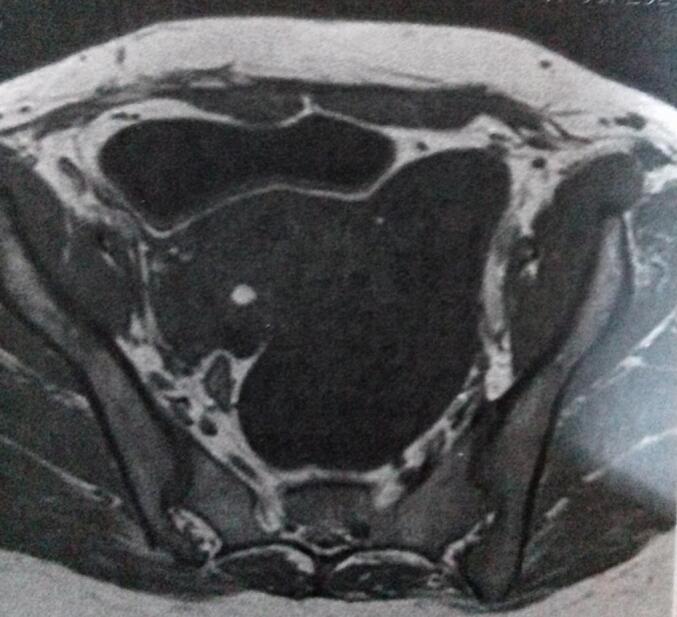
MRI demonstrating cystic lesion posterior to the urinary bladder in the left para-colic gutter.
